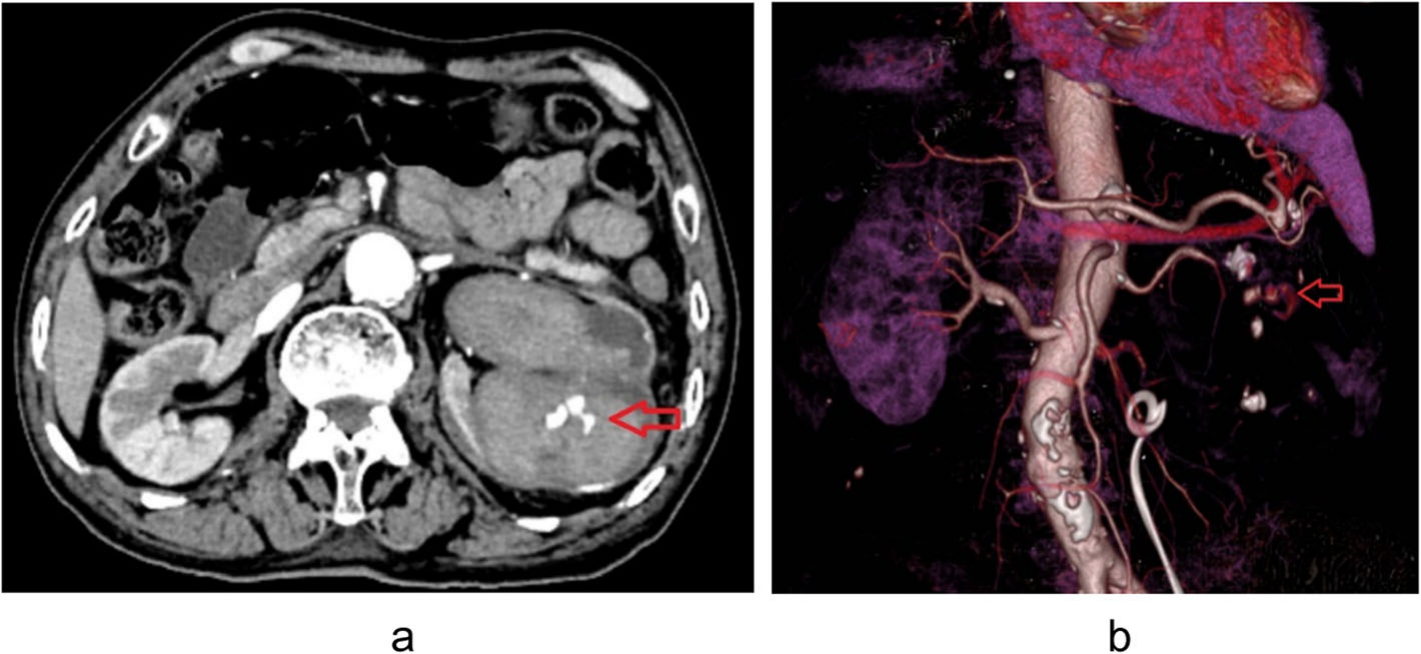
a multi-detector computed tomography angiography showed mild hydronephrosis with blood clots and AVF in the left kidney. b three-dimensional construction of renal arteries found an AVF
